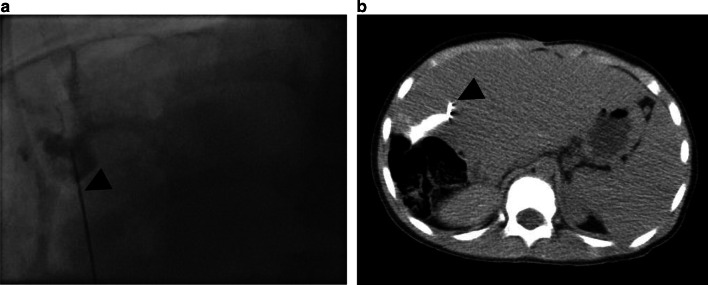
Percutaneous transhepatic puncture and sclerotherapy of the varices was performed. a Intraoperative radiograph. b Intraoperative computed tomography image. The black arrowhead indicates the needle puncture
